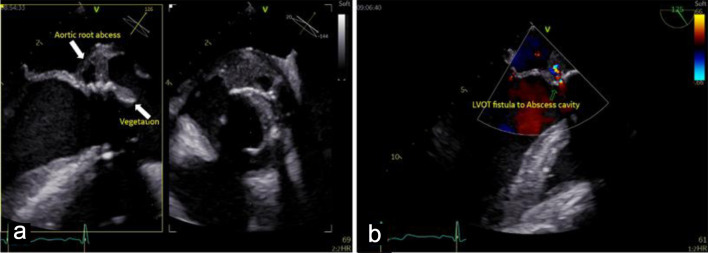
Mid-esophageal view transesophageal echocardiogram (TEE) demonstrates: (a) pedunculated vegetation (lower arrow, measured 3 × 6 mm) on the bioprosthetic aortic valve cusp, and paravalvular aortic-root abscess (upper arrow), indicating advanced infective endocarditis. (b) Doppler echocardiogram shows left ventricular outflow tract (LVOT) fistula to the abscess cavity.
